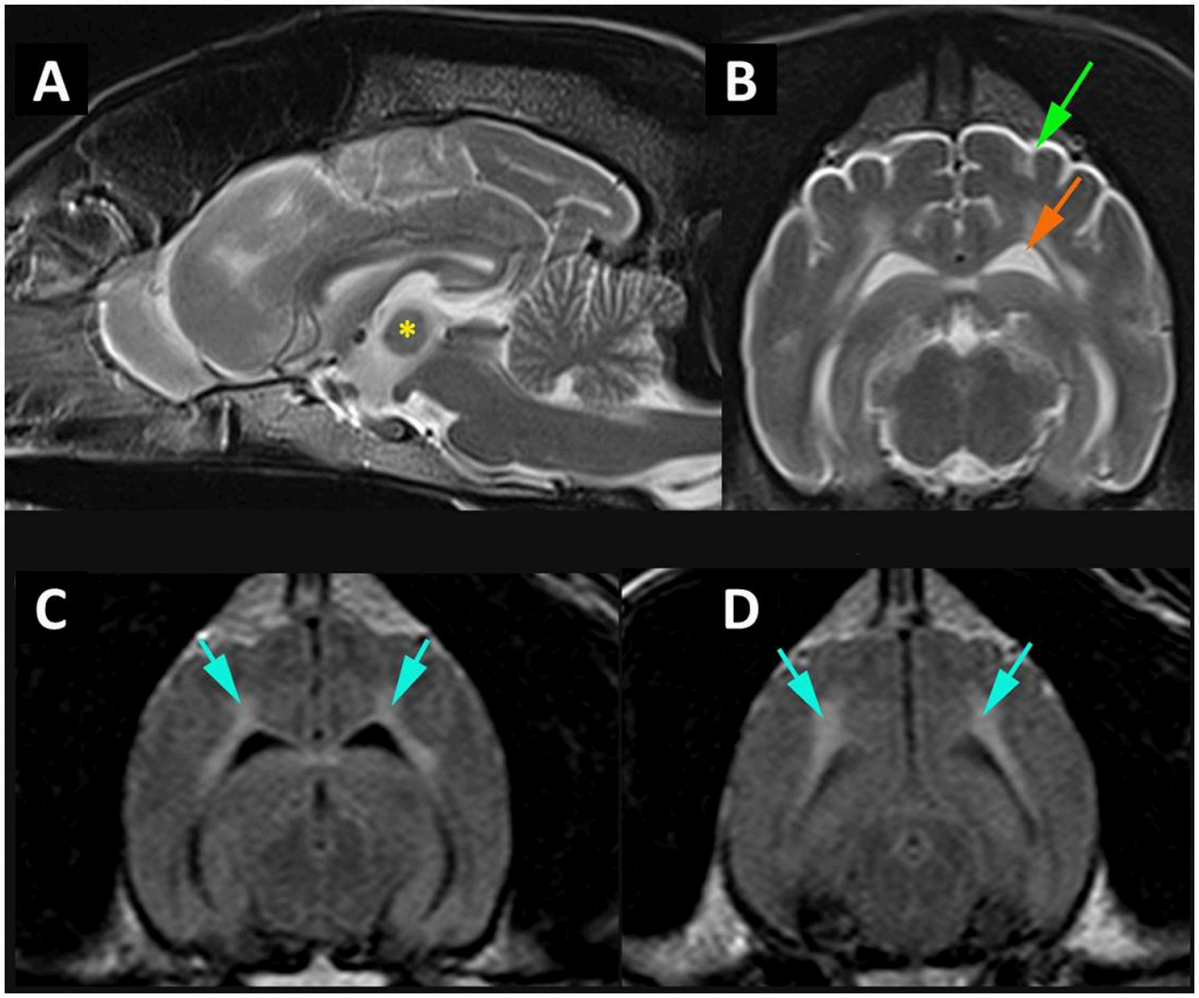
MR imaging of cortical atrophy and myelin degeneration. (A) Mid-sagittal T2W image of the brain demonstrating atrophy of the intra-thalamic adhesion (^*^) height 4.76 mm. (B) Transverse T2W at the level of temporal lobes demonstrating cortical atrophy with widening of the subarachnoid space (green arrow) and enlargement of the lateral ventricle (orange arrow). (C) Transverse FLAIR at level of temporal lobes and (D) transverse FLAIR at level of occipital lobes and midbrain demonstrating white matter hyperintensity (blue arrows).
